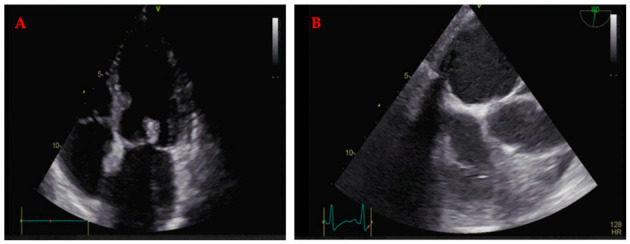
(A,B) Transthoracic ultrasound—four-chamber section, rearranged mitral valve, dilated left atrium (A). Transesophageal ultrasound—filamentous formation at the left atrium level, attached to the posterior face—aspect compatible with cor triatriatum sinister (B).
